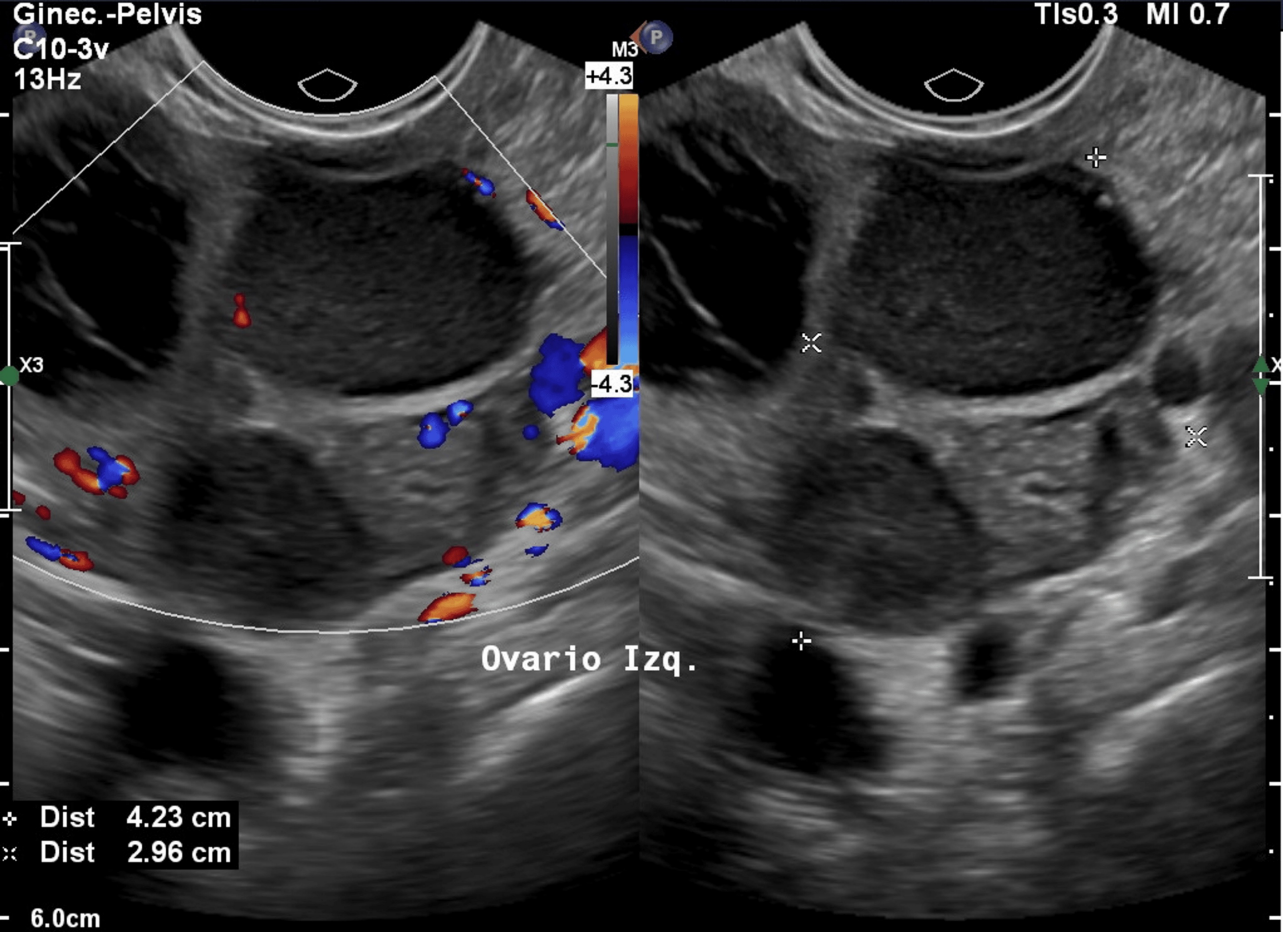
Transvaginal pelvic ultrasound demonstrating a predominantly solid left ovarian mass with heterogeneous echotexture and clearly defined margins.
